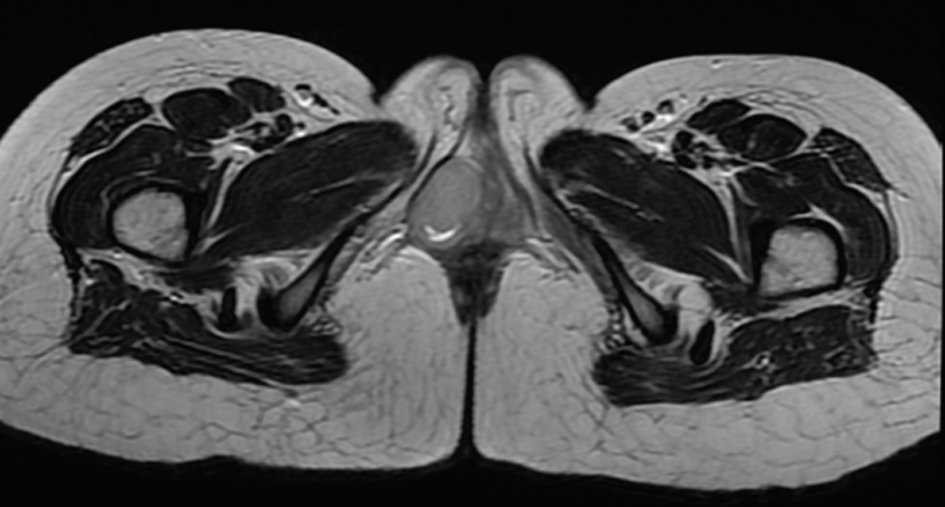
MRI axial T2WI-lesion appears hyperintense.
